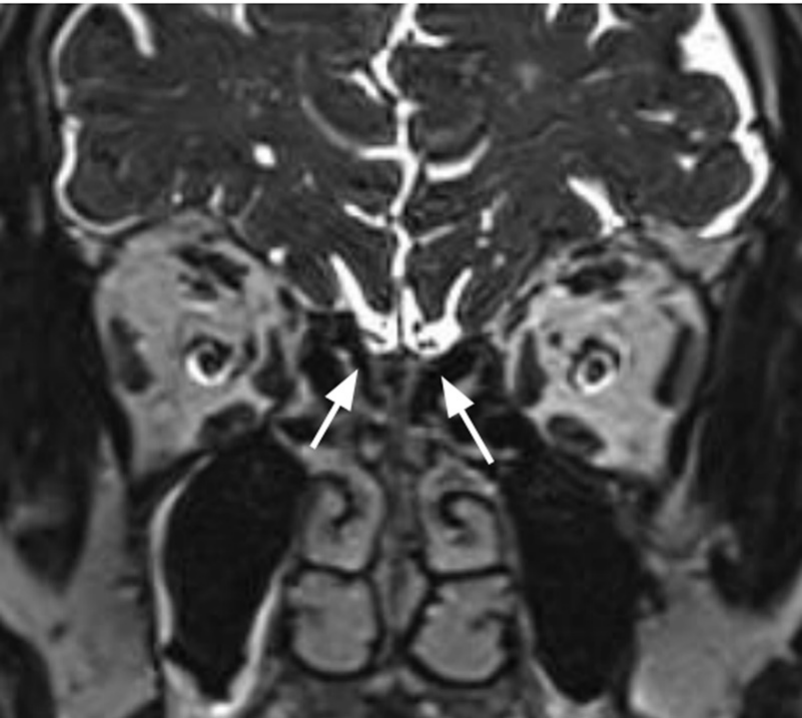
T2WI coronal MRI image of the present case report, with white arrow showing olfactory bulb mild atrophy (right 19.01 mm^3^; left 23.36 mm^3^).
